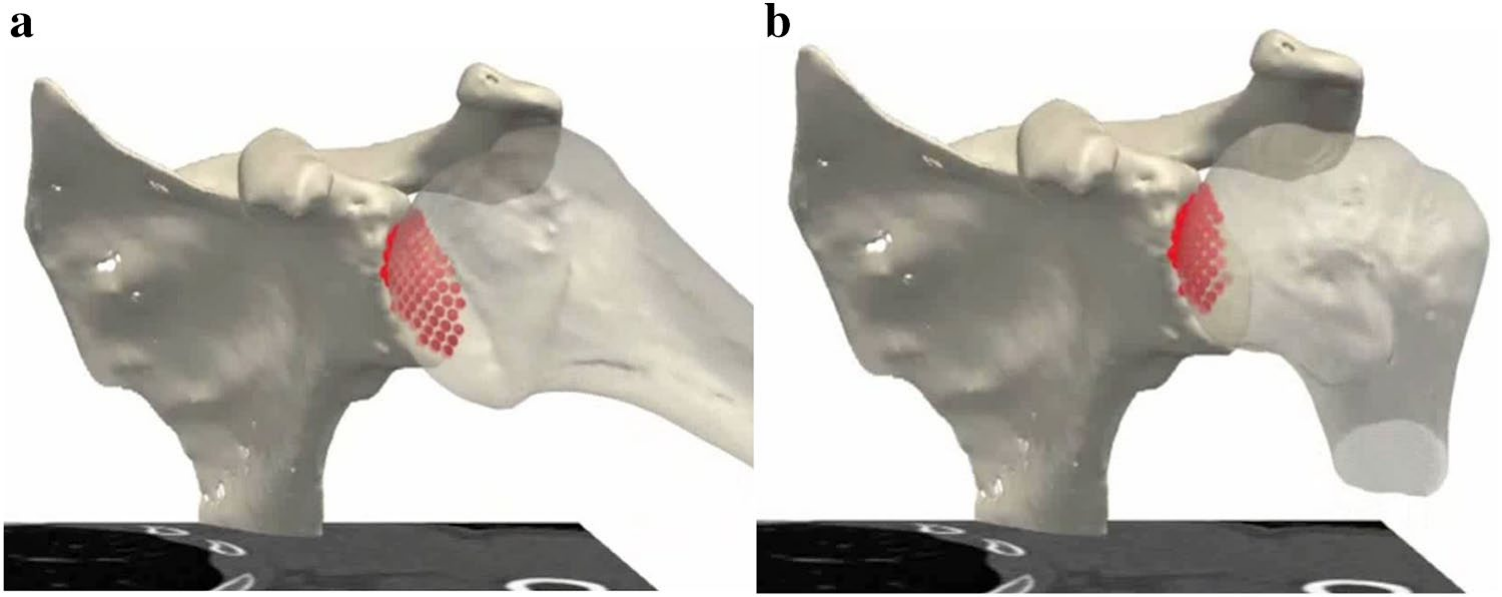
Dynamic CT scan. a, b Moving humerus with the measured contact area by the software (red dots) during different phases of movement
